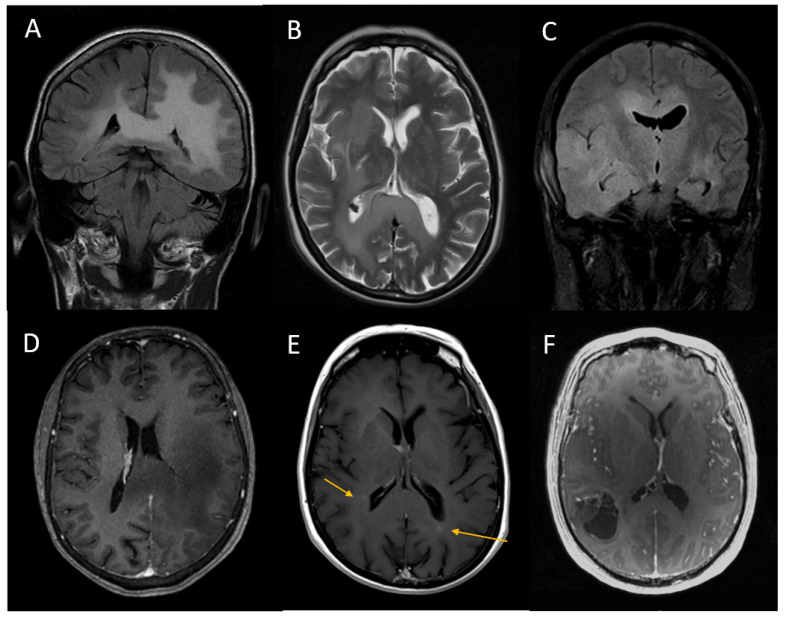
Fluid-attenuated inversion recovery (FLAIR, (A,C)), T2-weighted (B) and gadolinium-enhanced T1-weighted images (D–F) in three individuals with gliomatosis demonstrating non-enhancing (D), patchy solid enhancing ((E), arrows) and rim-enhancing (F) infiltration.
